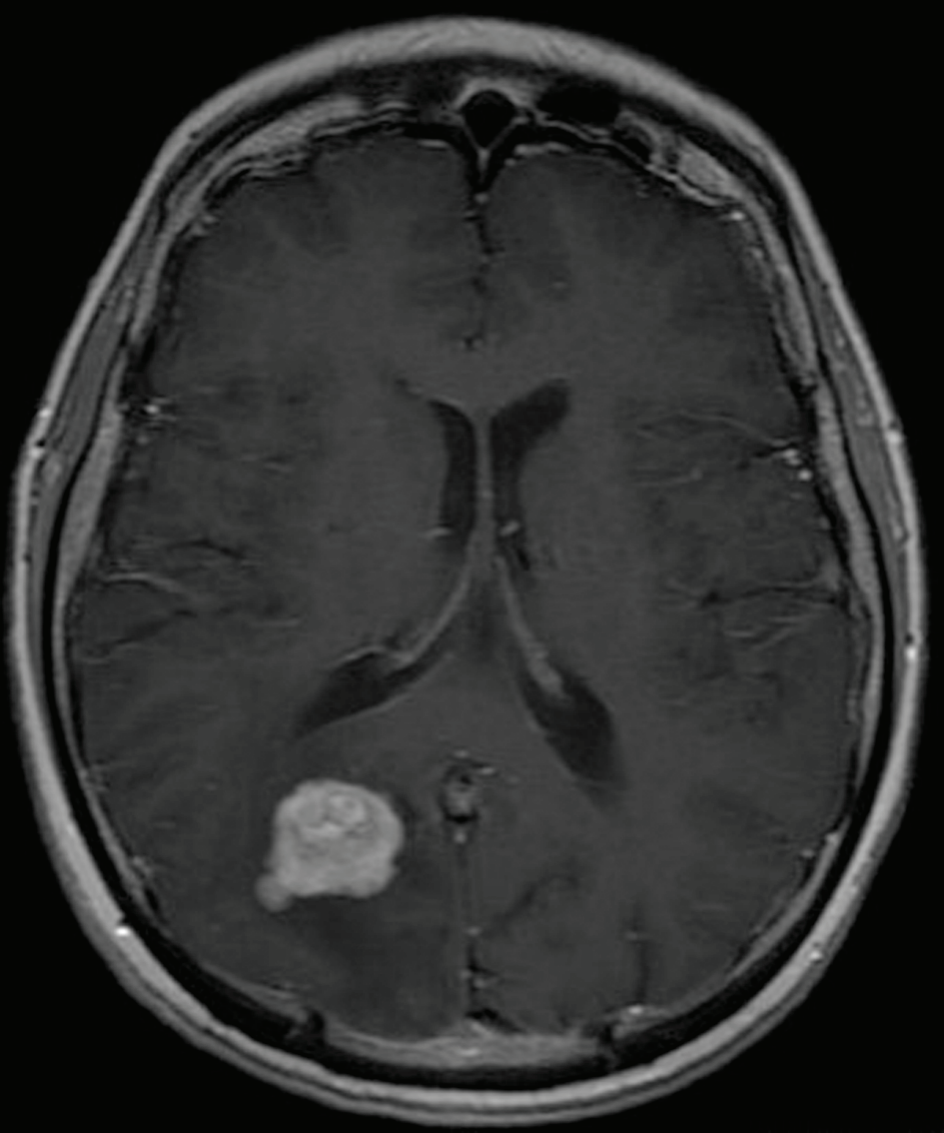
Pre-operative brain MRI T1 sequence with contrast gadolinium which shows the enhancing of the tumor.
